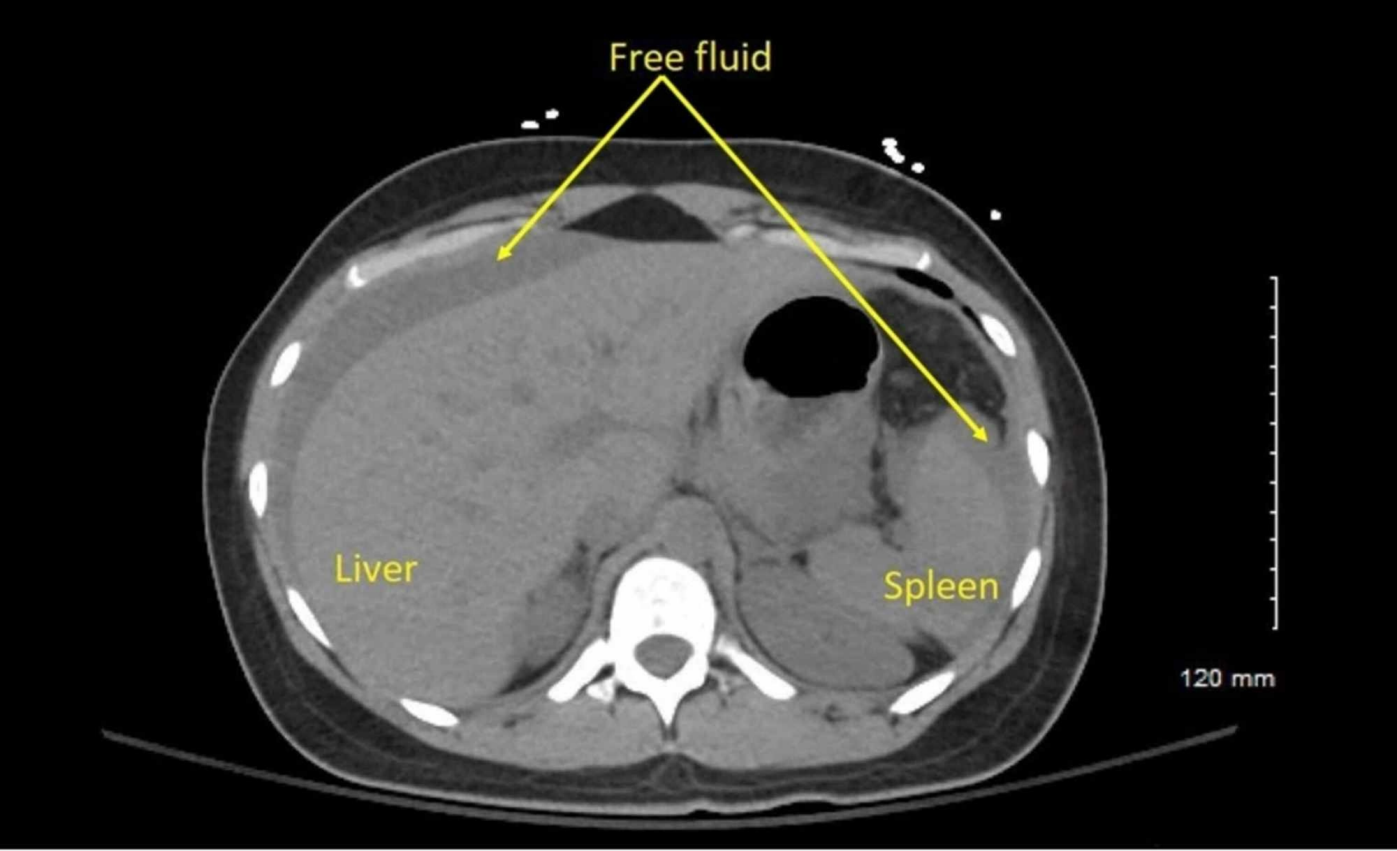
Transverse CT at level of mid-spleen with intraperitoneal free fluid.
